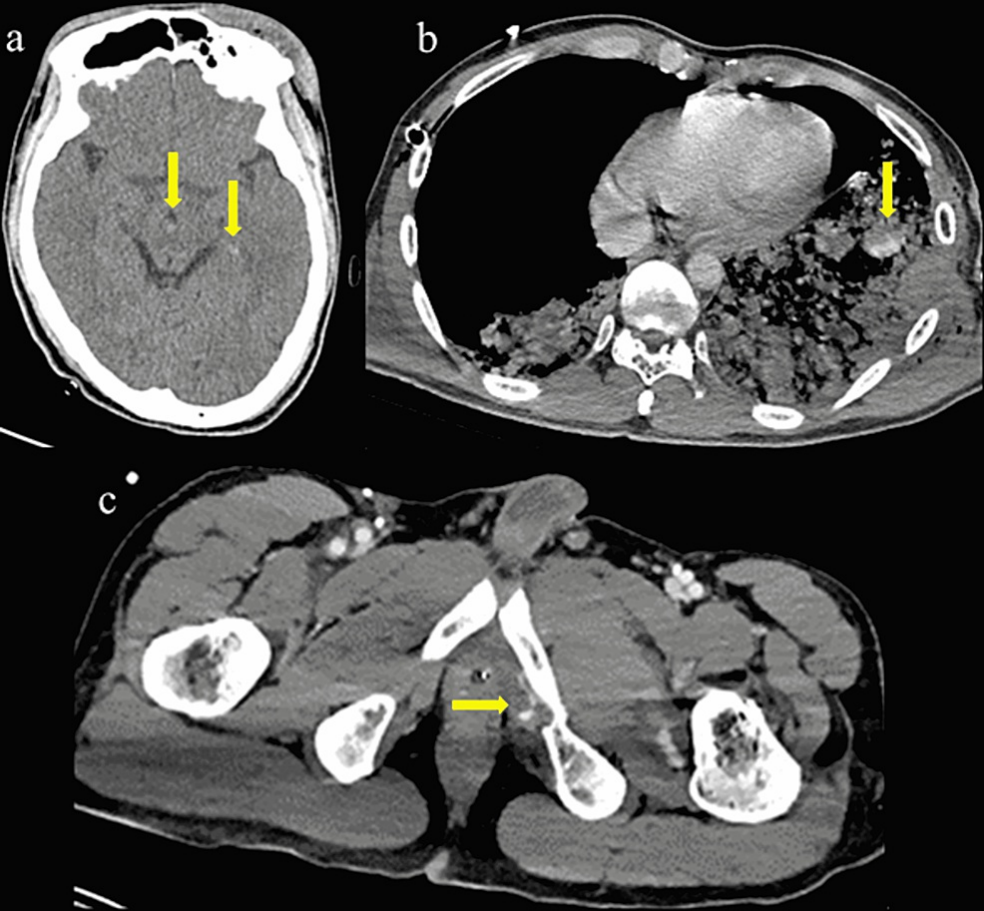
CT findings on admission (a) Head CT showing brainstem hemorrhage and traumatic subarachnoid hemorrhage (AIS code: 140210.5). (b) Chest CT showing active bleeding in the thoracic cavity. (AIS code: 441456.5). (c) Pelvic fracture and active bleeding around the fracture (AIS code 852608.4).
